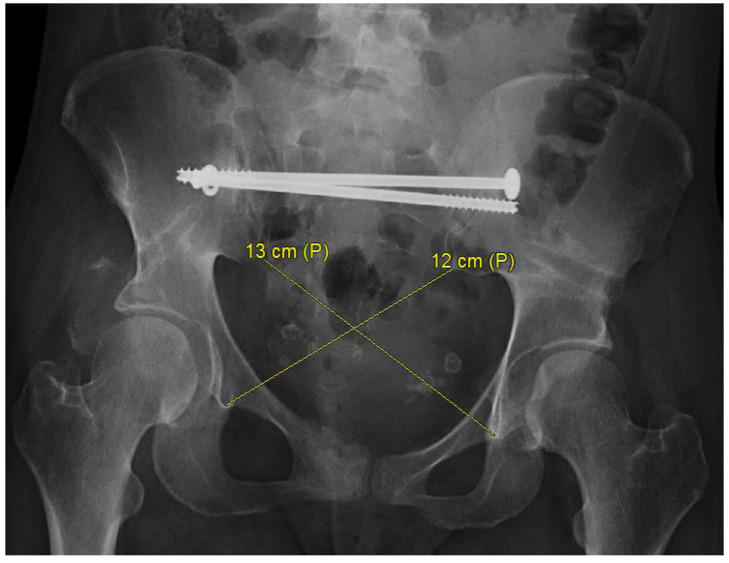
Anteroposterior radiograph of a pelvic malunion with internal rotation deformity of the right hemipelvis demonstrating diagonal distance from the low border of the sacroiliac joint to the bottom of the radiographic tear drop in skeletally mature patients.
